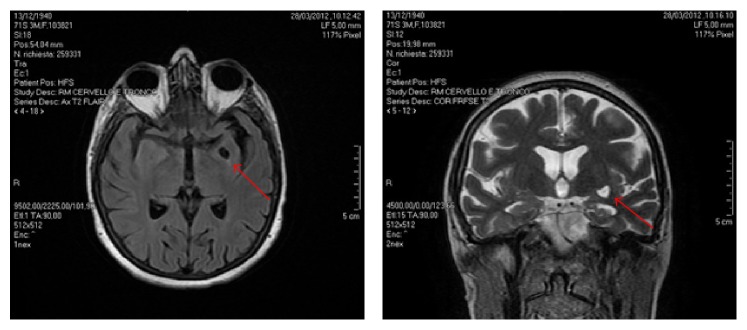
An expanded round cavity with liquid content and thin halo of gliosis was evidenced with MRI (indicated with arrows).
